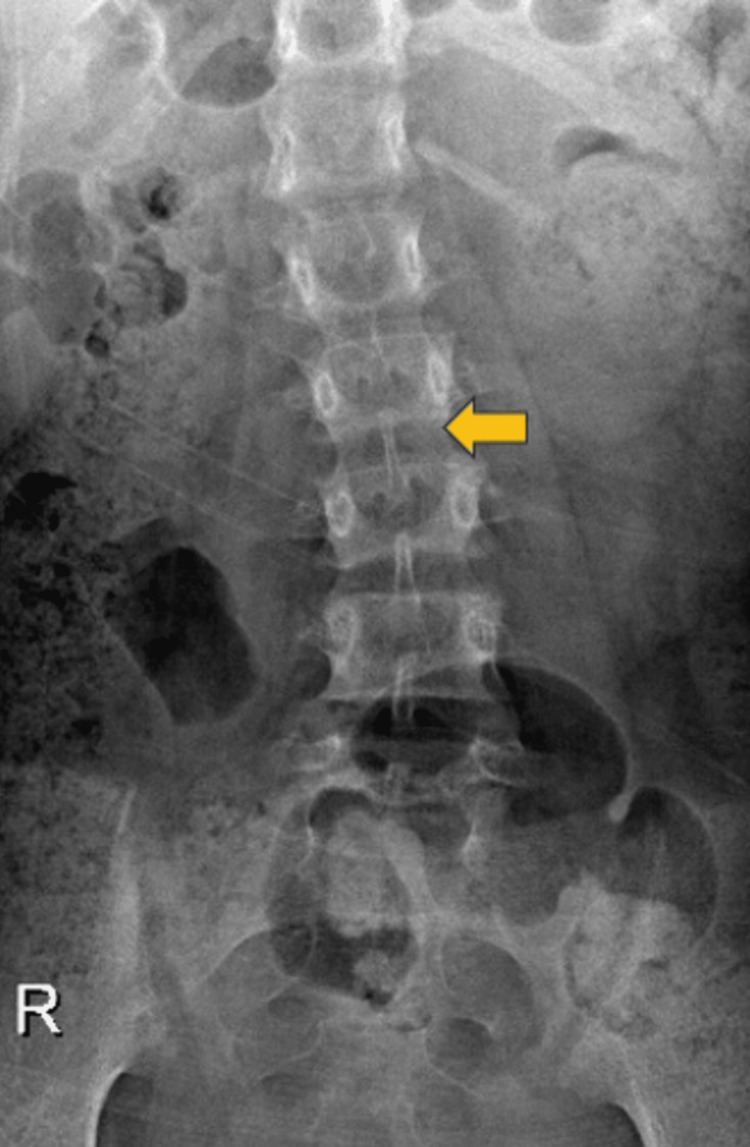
A radiograph (anteroposterior view) of the lumbar spine shows levoconvex scoliosis (yellow arrow).
